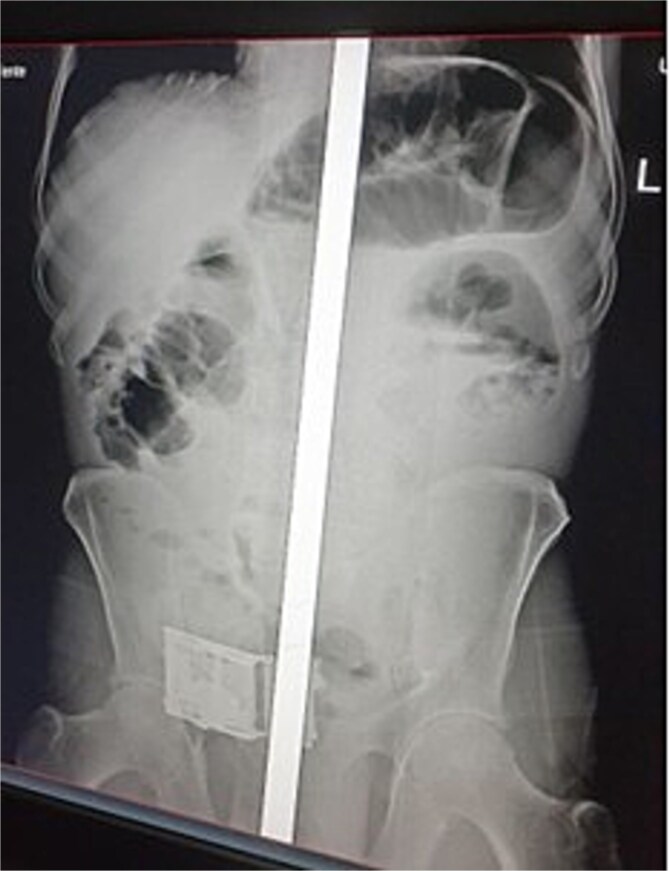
Abdominal radiograph showing multiple distended loops of small bowel.
